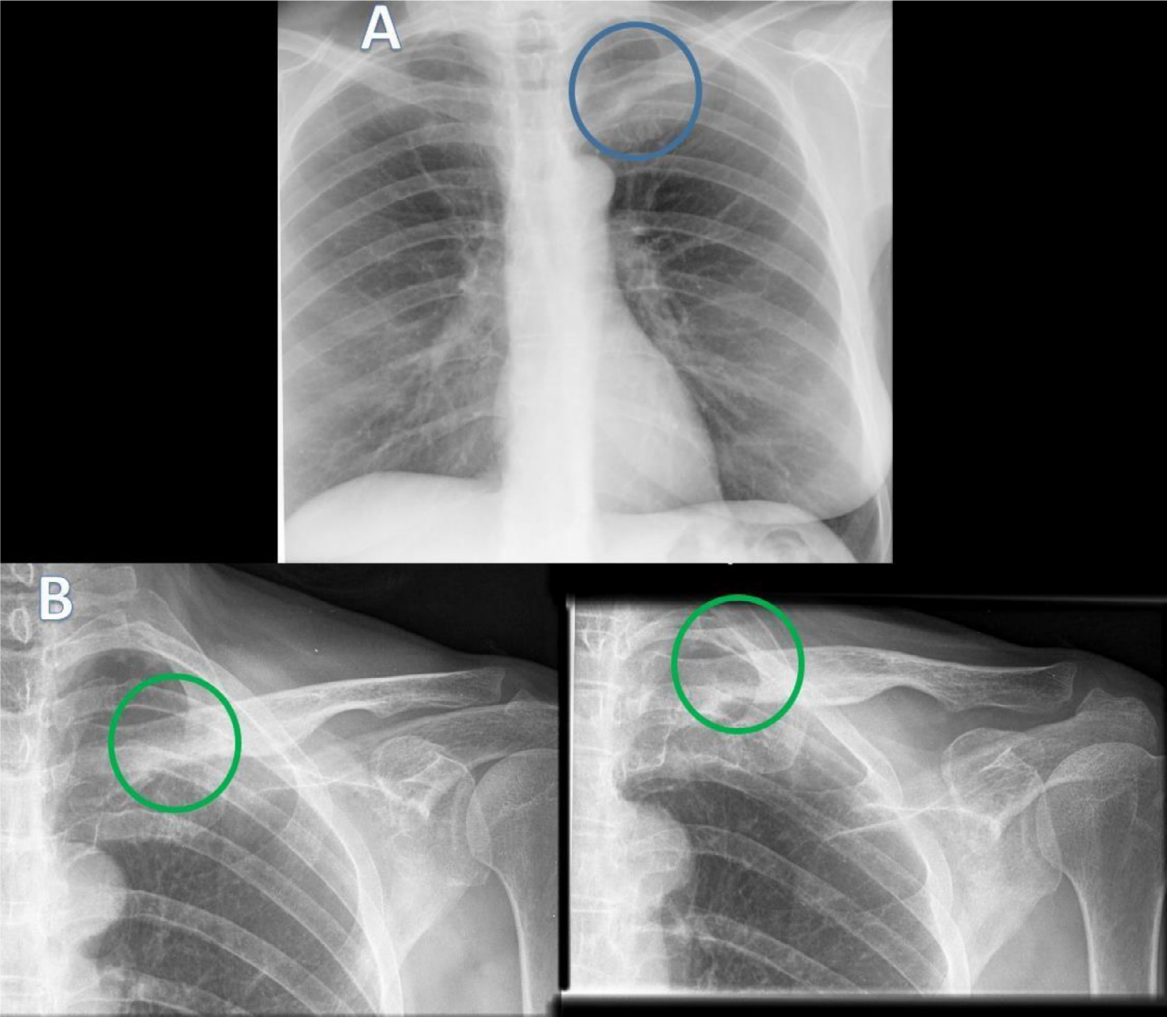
Standard chest radiograph in the posterior-anterior view (A) and plain X-ray film of the left clavicle (B) showing increased opacification at the left medial clavicular region (blue circle). The plain X-ray film of the left clavicle shows concentric thickening of the medial third and middle third of the left clavicle without involving the cortex (green circles).
